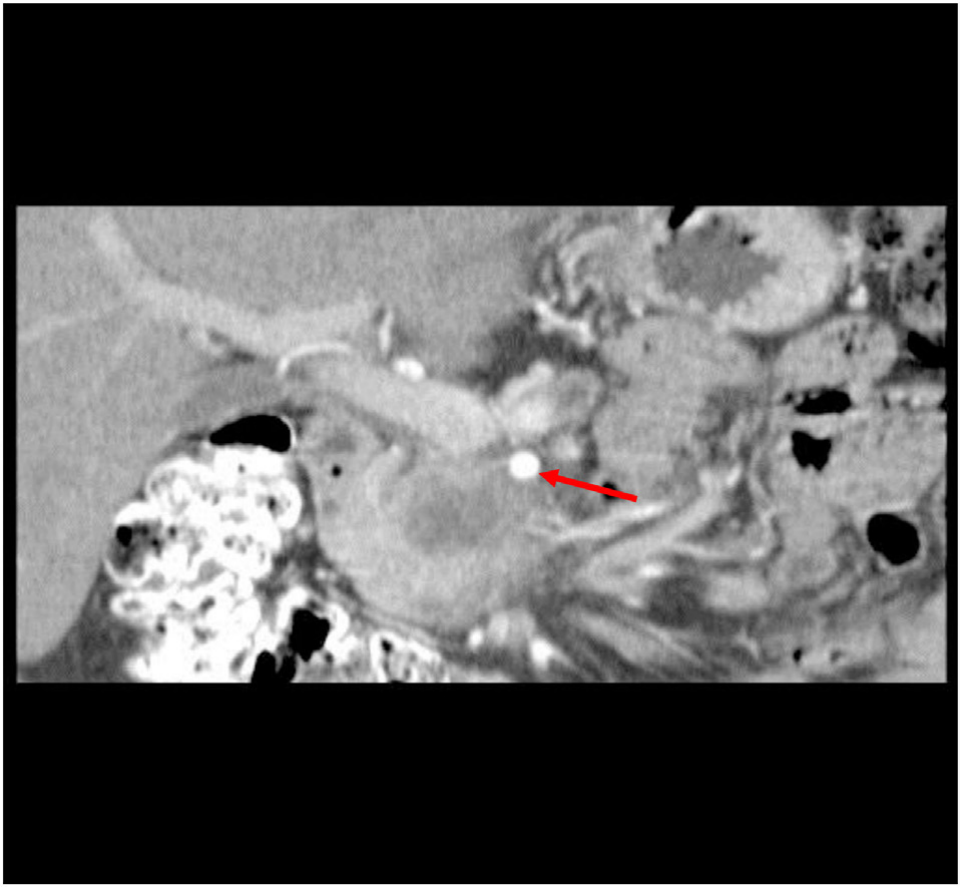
CT abdomen at restaging following neoadjuvant chemo-radiation therapy showed a 3.3 cm × 3.1 cm mass, decreased from initial size with involvement of the SMA (see arrow) and SMV improved.
